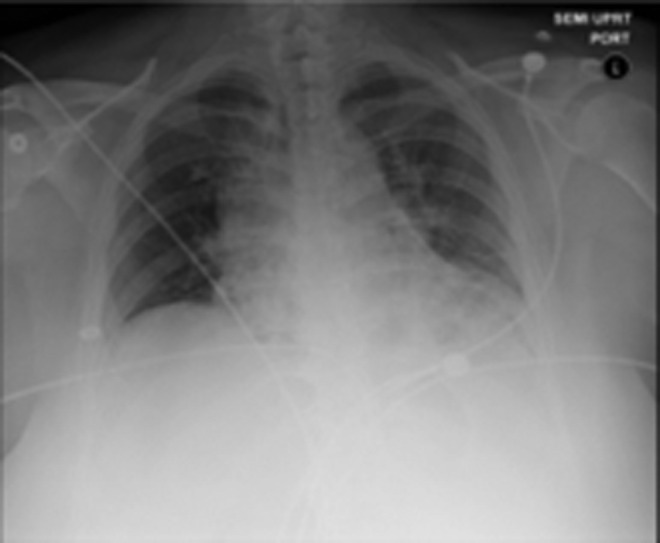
Postoperative chest X-ray performed immediately postoperatively demonstrated no evidence of intrathoracic fluid collection.
